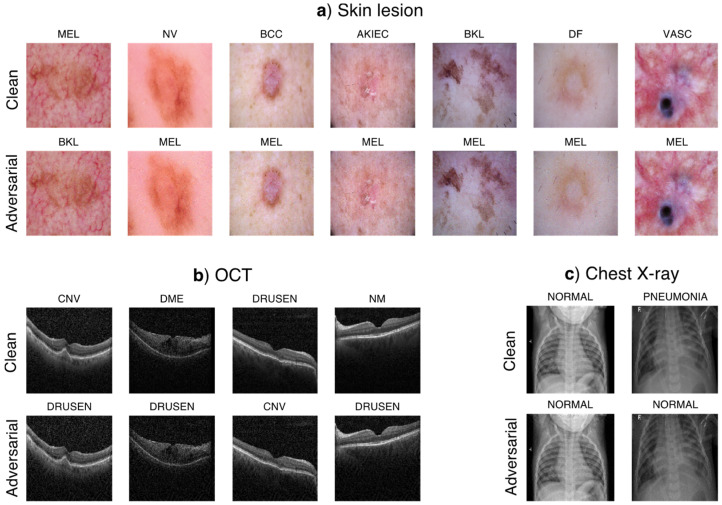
Clean images and their adversarial examples generated using non-targeted UAPs from the ImageNet dataset, against the Inception V3 model for the skin lesion (a), OCT (b), and chest X-ray (c) image classifications. ζ=4% in (a,c) and ζ=8% in (b). Labels next to the images are the predicted classes. The clean (original) images are correctly classified into their actual labels.
